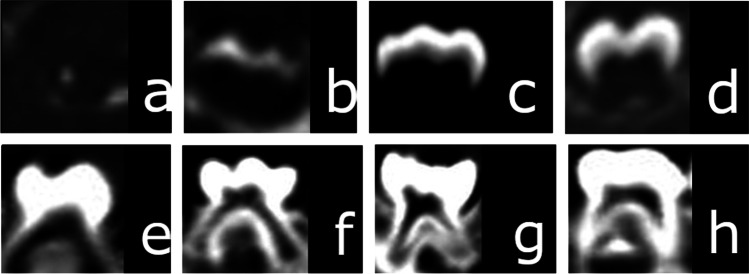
Computed tomography scans showing deciduous tooth maturation: A Stage 1 – beginning of calcification, calcification of single occlusal points. B Stage 2 – calcification points fuse to form the occlusal surface. C Stage 3 – dentin is visible. D Stage 4 – beginning of root formation, a dentine spicule. E Stage 5 – root length remains shorter than crown height. F Stage 6 – full root length has not been reached, canals are divergent in the apical area. G Stage 7 – root length is nearly full, apical canal walls are parallel or slightly convergent. H Stage 8 – completely mature root
